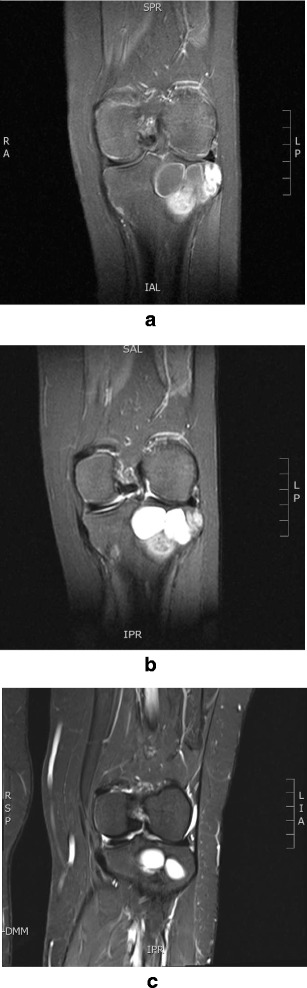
Magnetic resonance imagining of the patient’s left knee showing a heterogeneous, solid, and soft tissue mass involving the proximal epimetaphysis of the tibia and extending to the subarticular cortex. a Preoperative scan. b Scan obtained 6 months postoperatively showing a mild decrease in the size of the lesion with increased sclerosis of the solid component. c Scan obtained 2.5 years postoperatively showing a further decrease in the size of the mass
